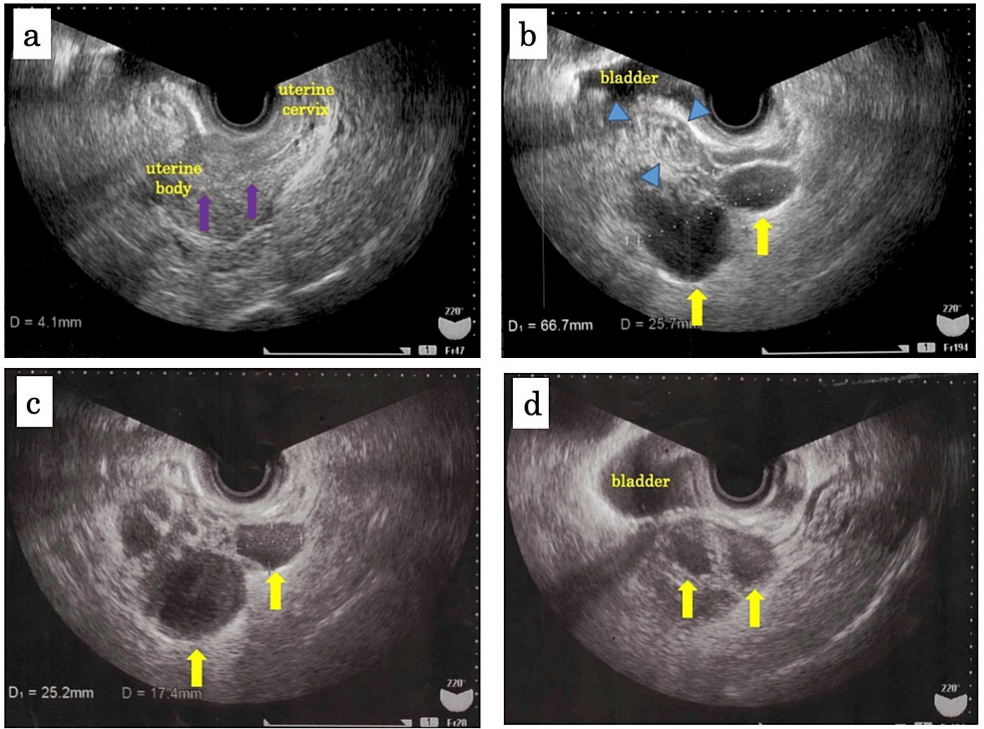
Transvaginal sonography on admission (a-b), on day 7 (c), and on day 18 (d) after admission (a, b) Transvaginal sonography on admission showed the endometrium (4.1 mm) was not thickened (purple arrows), the left ovary was not enlarged (blue arrows), and the left oviduct was enlarged and distorted resembling consecutive cysts (yellow allows); (c) Transvaginal sonography on day 7 after admission showed no significant change in the left oviduct (yellow allows); (d) Transvaginal sonography on day 18 after admission showed that the left oviduct had been smaller than at admission (yellow allows).
